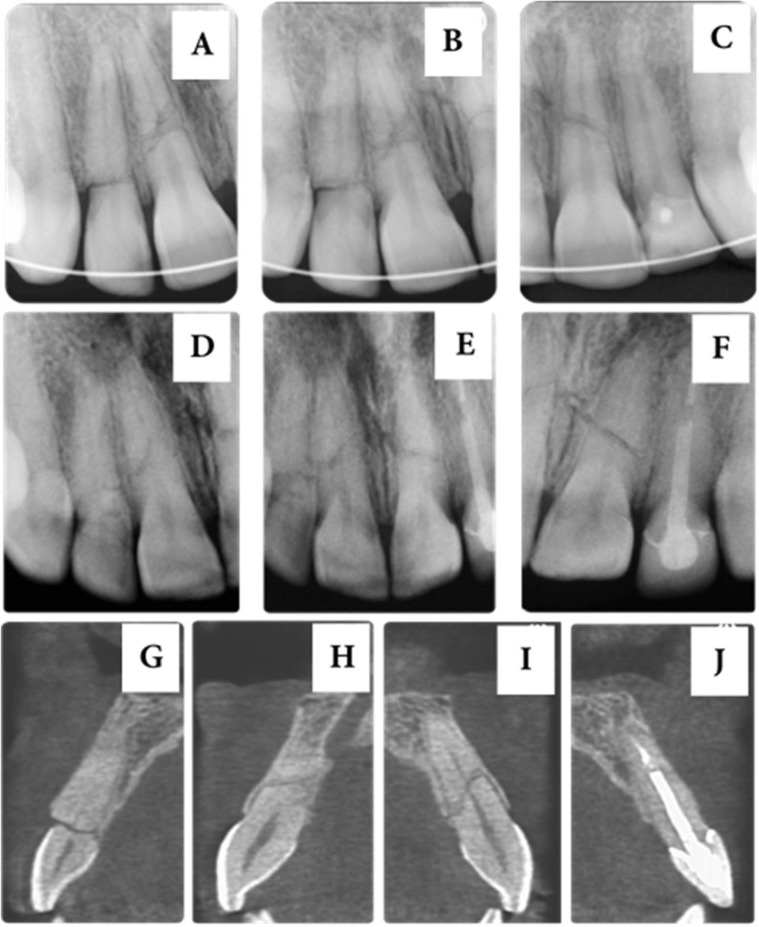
A to C) *Periapical radiographs acquired during initial care, demonstrating horizontal fractures in the cervical third of the right maxillary lateral incisor, and middle third of the right maxillary central incisors, and coronary fracture of the left maxillary lateral incisor*; D to F) *Periapical radiographs acquired 13 years after the initial treatment suggesting the formation of mineralized tissue between the fragments of the maxillary central incisors and the right maxillary lateral incisor*; G to J) *Computed tomographic images suggesting an aspect of normality for each fractured incisor*
